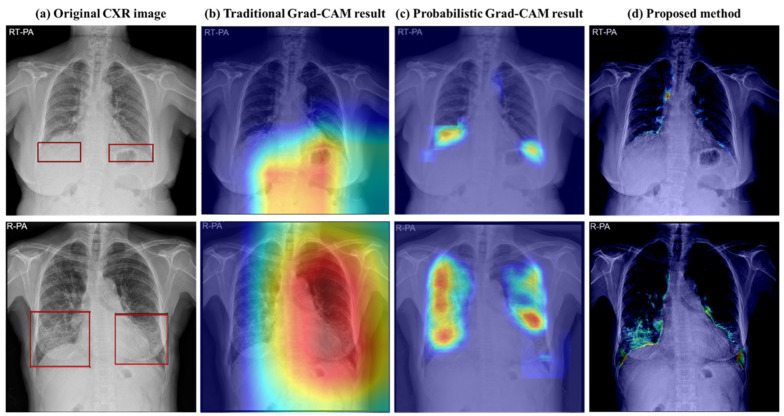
Results of Grad-CAM method and image-to-image translation method: (a) original CXR image: the original chest X-ray of a patient with ILD, and the red box indicates the abnormal area; (b) traditional Grad-CAM results: images with abnormal areas are highlighted using Grad-CAM; (c) probabilistic Grad-CAM results: images with probabilistic Grad-CAM were used for more detailed area analysis; (d) proposed method (image-to-image translation method) results: analyzing the images using the method proposed in this study, the ILD areas were accurately displayed in pixel units compared to Grad-CAM.
